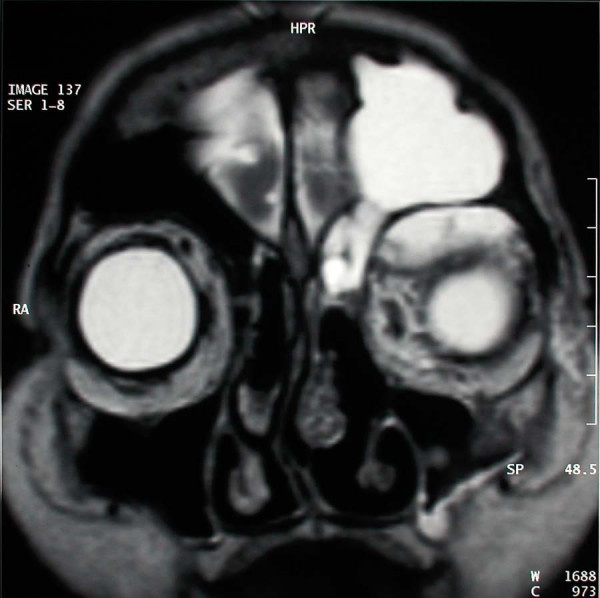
Coronal magnetic resonance imaging demonstrating a frontal mucocele and an orbital cystic mass.
